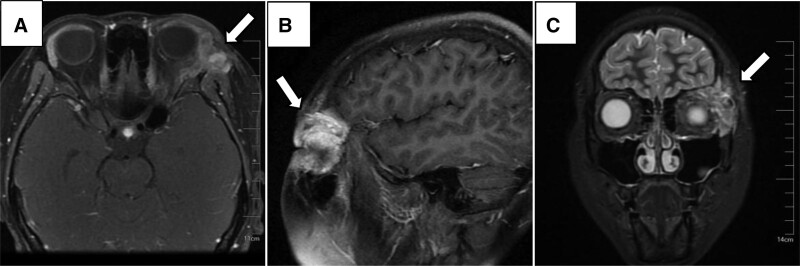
MRI from axial (A), sagittal (B), and coronal (C) views revealed an enhanced tumor on the lateral side of the left orbit involving the left orbital soft tissue, left eyelid, temporalis muscle, and left zygomatic arch. MRI = magnetic resonance imaging.
